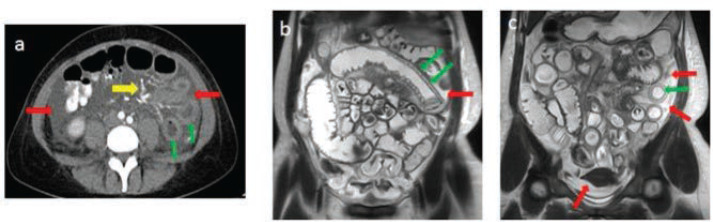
Abdominal CT and MR images of patient 3. (a) Axial CTA image; Ascites (red arrows); wall thickening of jejunal arterial branches (yellow arrow); jejunal wall thickening (green arrows). (b) MRI T2 haste coronal image: Jejunal wall thickening (green arrows); ascites (red arrow). (c) MRI T2 haste coronal image: Jejunal wall thickening (green arrow); ascites (red arrows).
